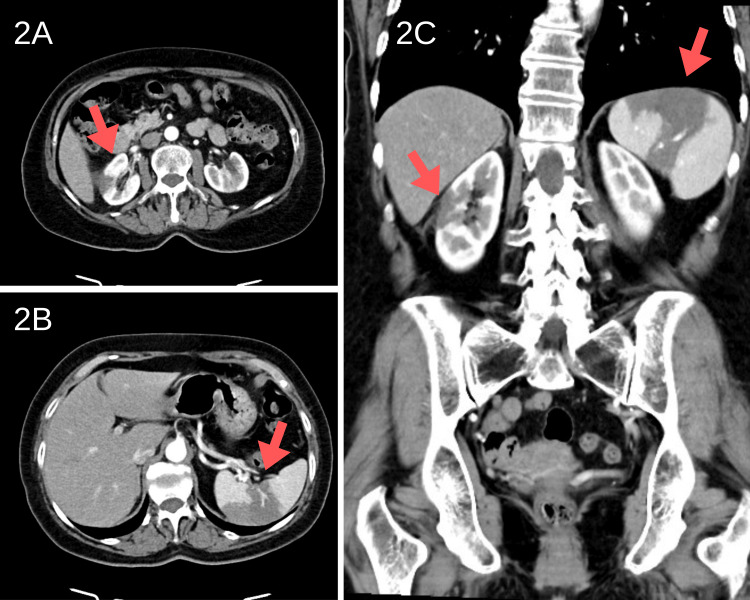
Contrast-enhanced abdominal CT images demonstrating renal and splenic infarctions. (2A) Axial image showing a wedge-shaped non-enhancing hypodense area in the lateral mid-portion of the right kidney, representing a perfusion defect consistent with renal infarction (arrow). (2B) Axial image showing a non-enhancing hypodense area in the spleen, consistent with splenic infarction (arrow). (2C) Coronal reconstruction demonstrating simultaneous right renal and splenic perfusion defects. Red arrows indicate the infarcted areas.
